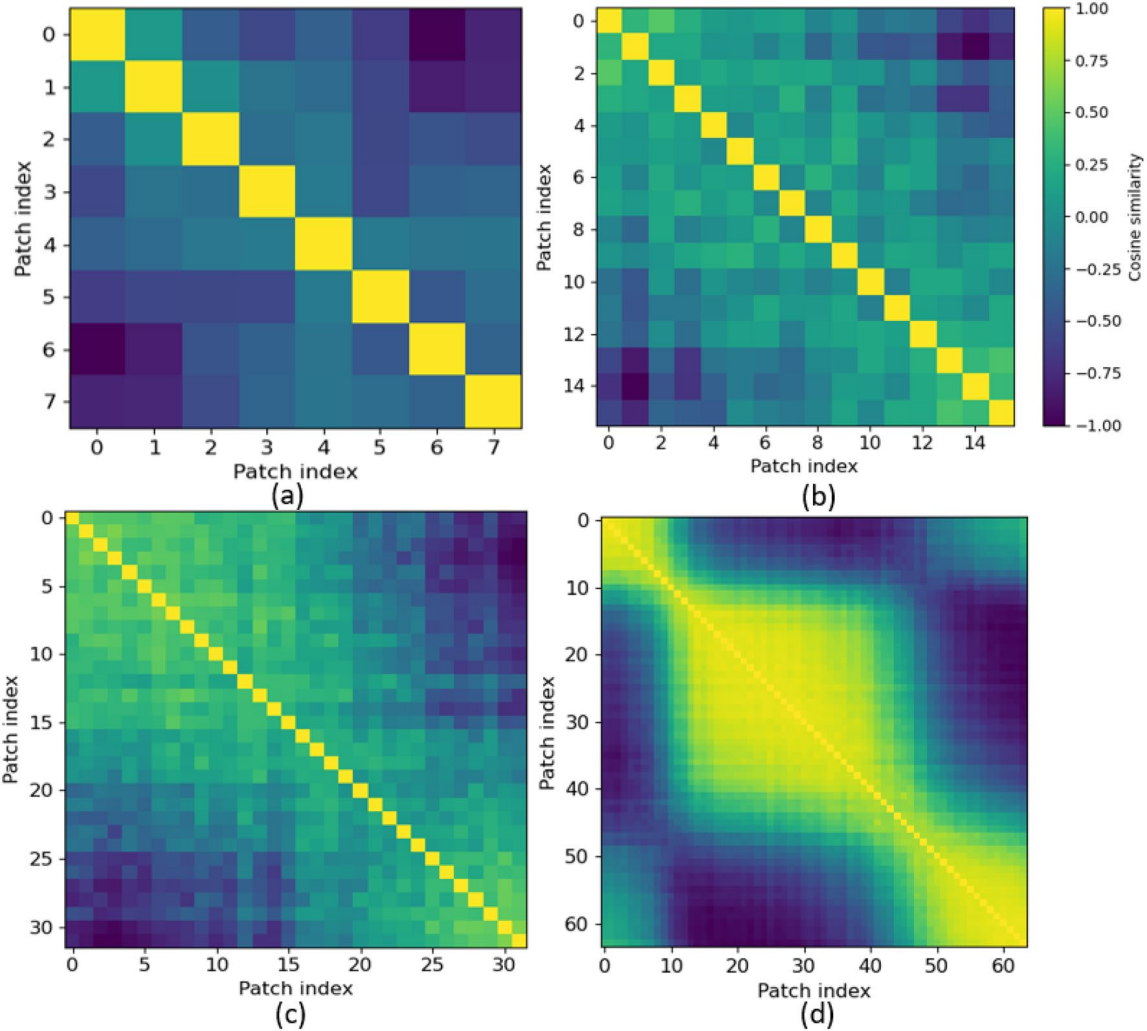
Cosine similarities of repetition 3, subject 20 of $$\text {CT-HGR}$$-V2 for (a) $$W=64$$ (b) $$W=128$$ (c) $$W=256$$ and (d) $$W=512$$.
